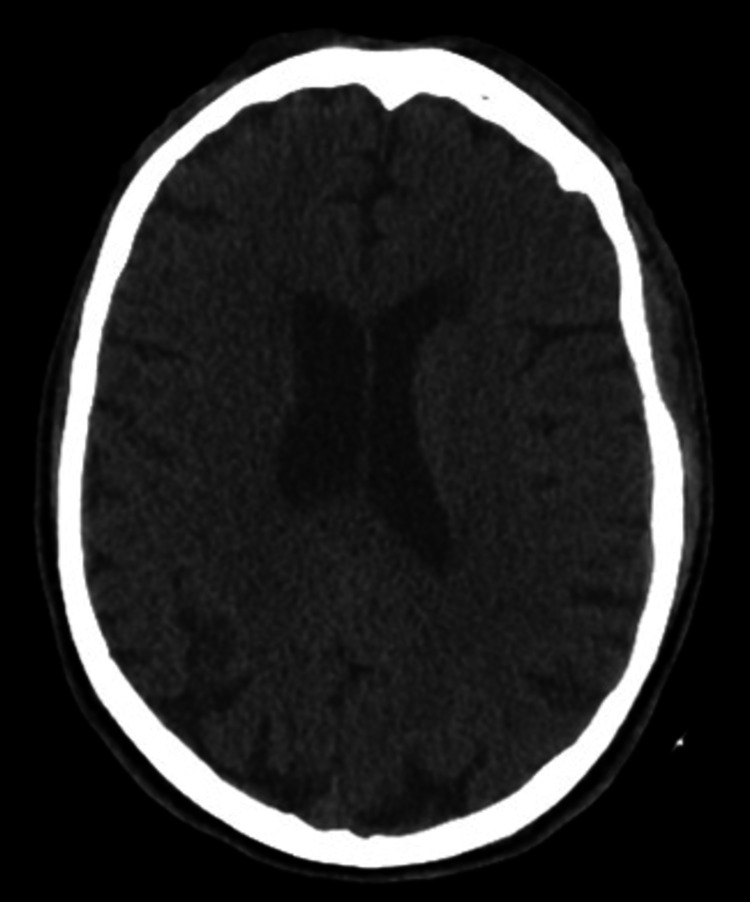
Normal head computerized tomography (CT) scan
